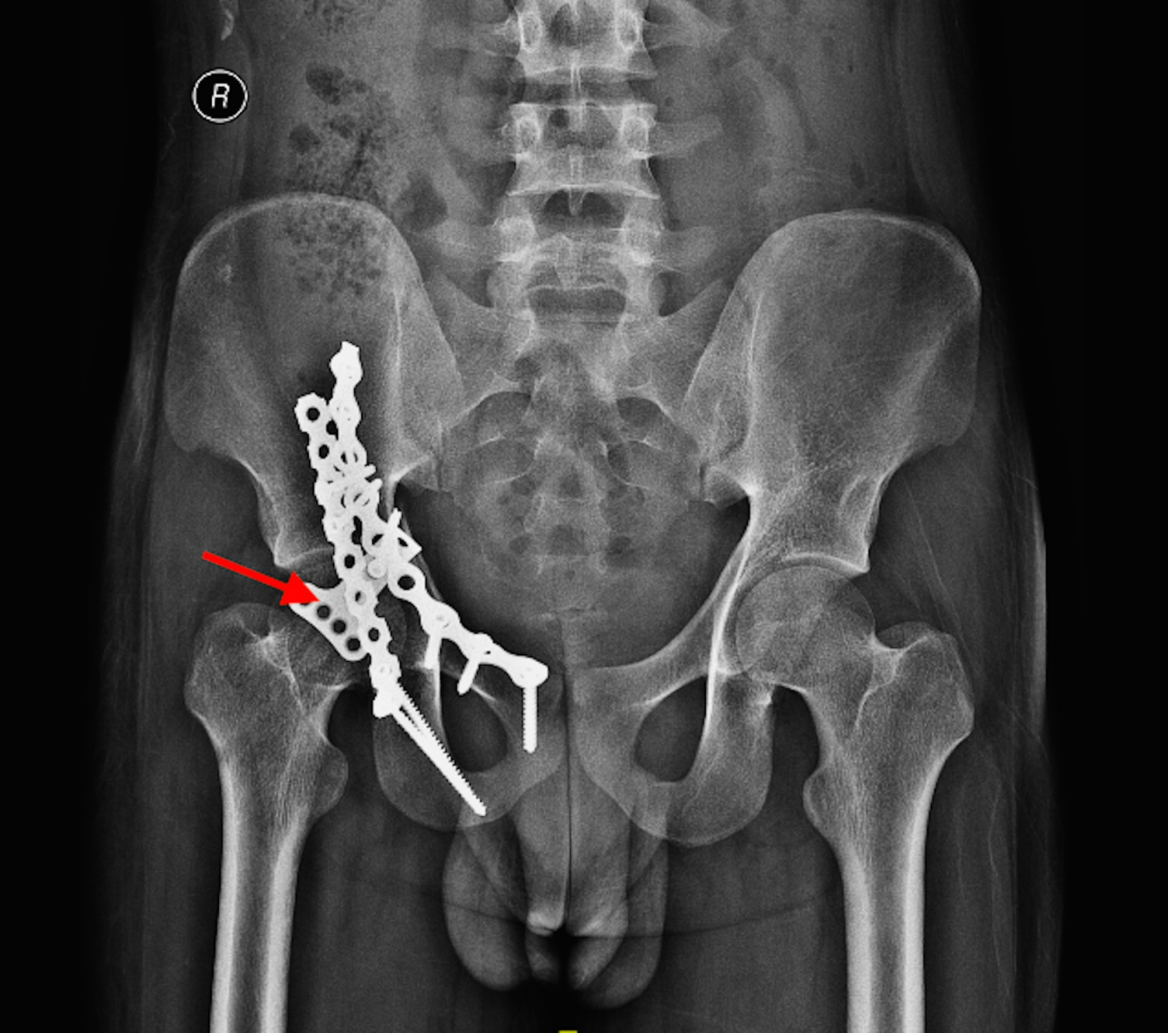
X-ray pelvis shows the right acetabulum fixed internally with plates and screws (marked by arrow)
